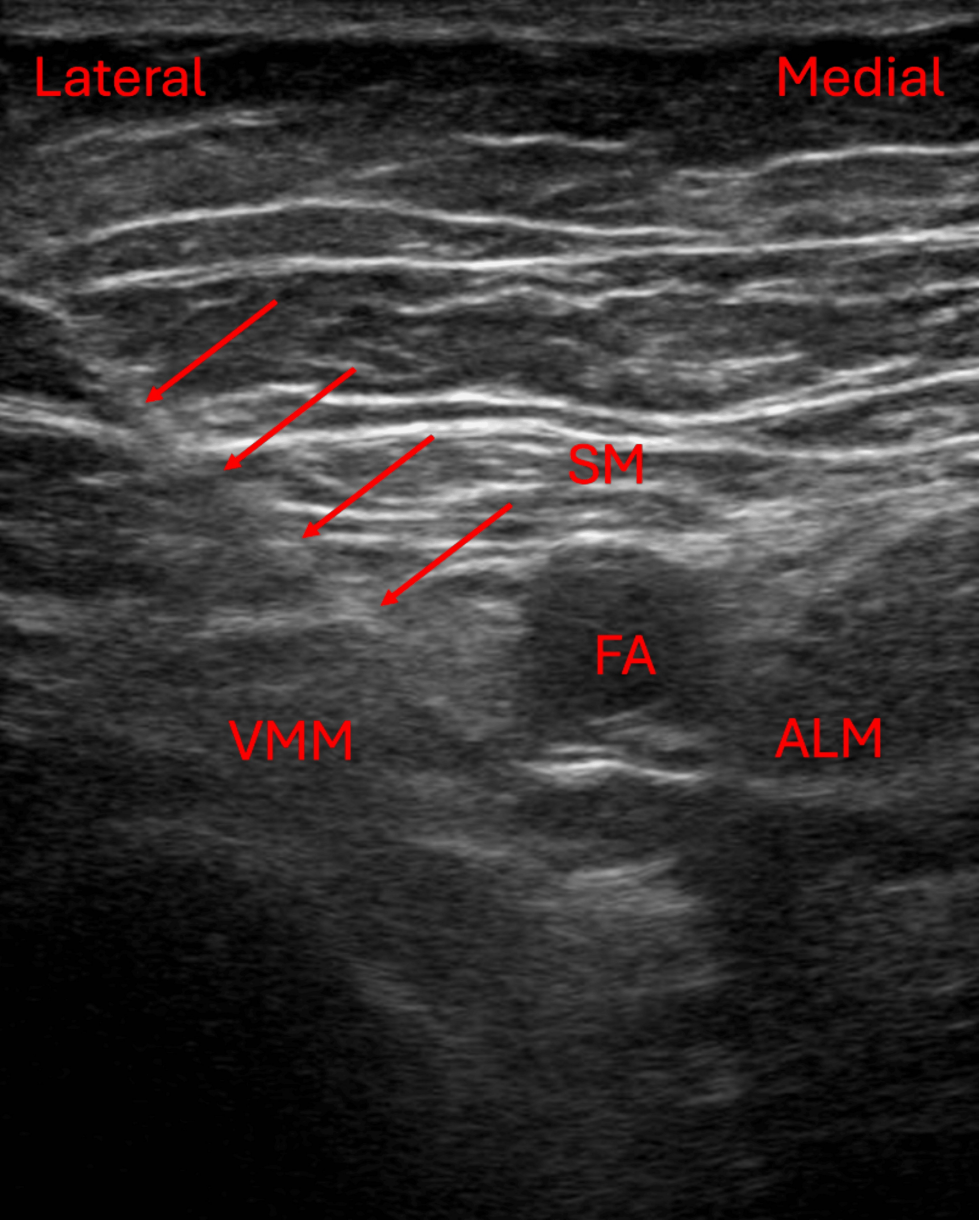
Ultrasound image of this patient's adductor canal block including the needle trajectory (arrows) with the tip of the needle positioned just lateral to the femoral artery within the adductor canal. The femoral artery (FA) courses through the adductor canal, which is bordered by the sartorius muscle (SM) anteriorly, the vastus medialis muscle (VMM) anterolaterally, and the adductor longus muscle (ALM) posteromedially.
